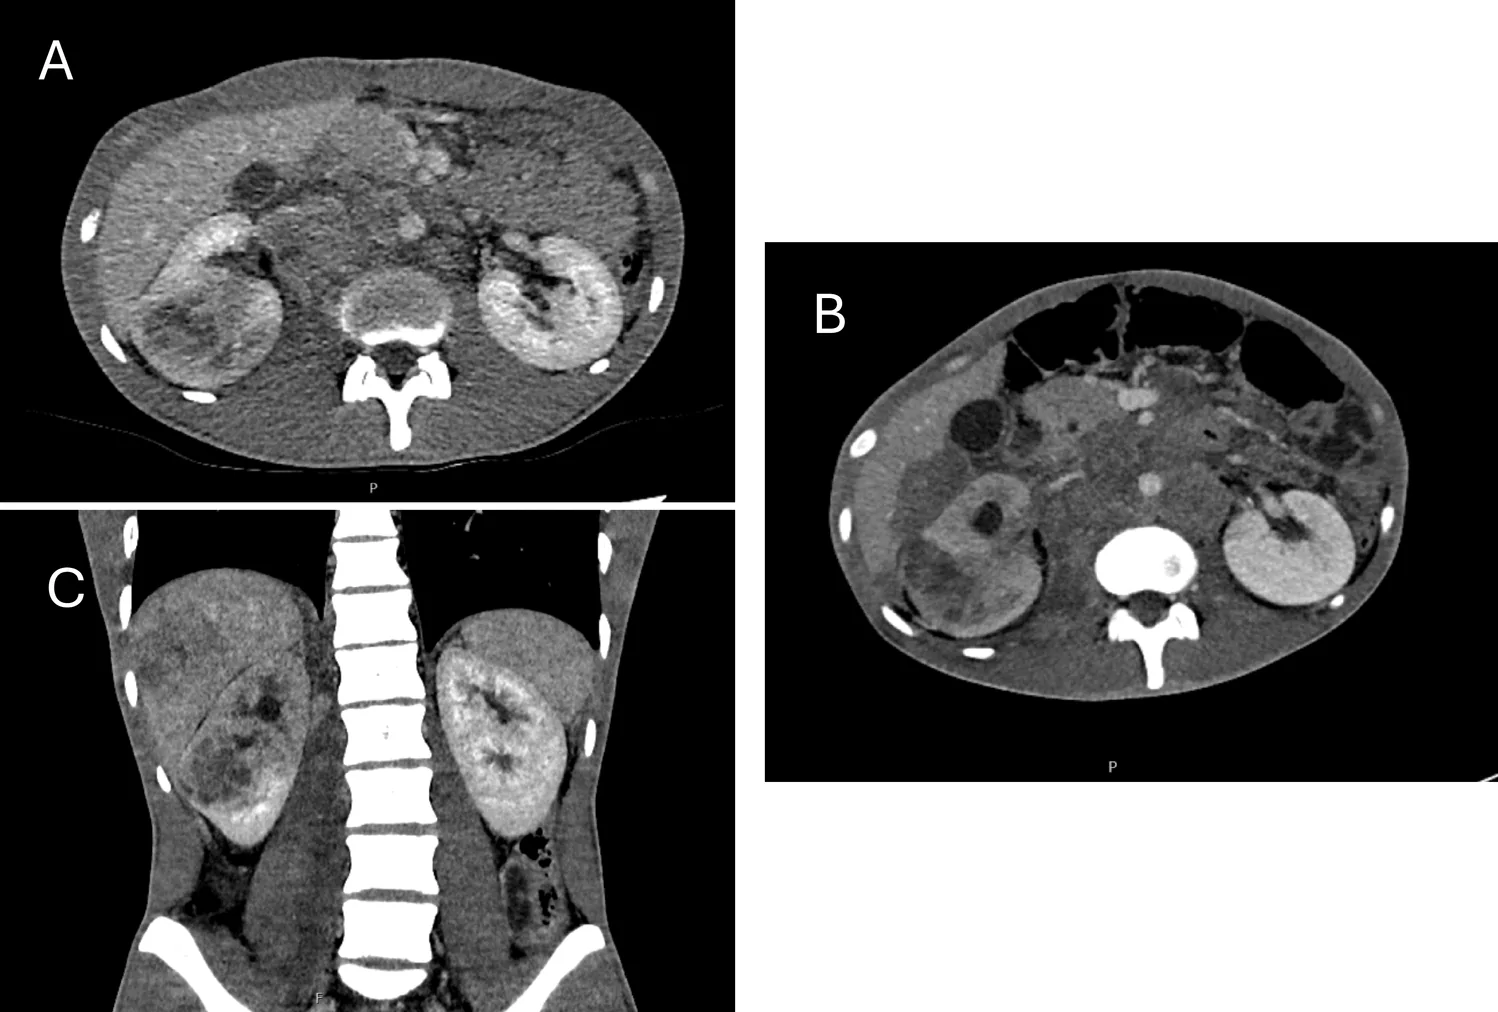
22-year-old Black male without sickle cell trait presenting with abdominal pain. A, B Axial and C coronal contrast-enhanced venous-phase CT scans show a largely necrotic right renal tumour with upper pole caliectasis, retroperitoneal lymph nodes encasing the aorta and inferior vena cava, and a liver metastasis. Lung metastases were also present (not shown)
